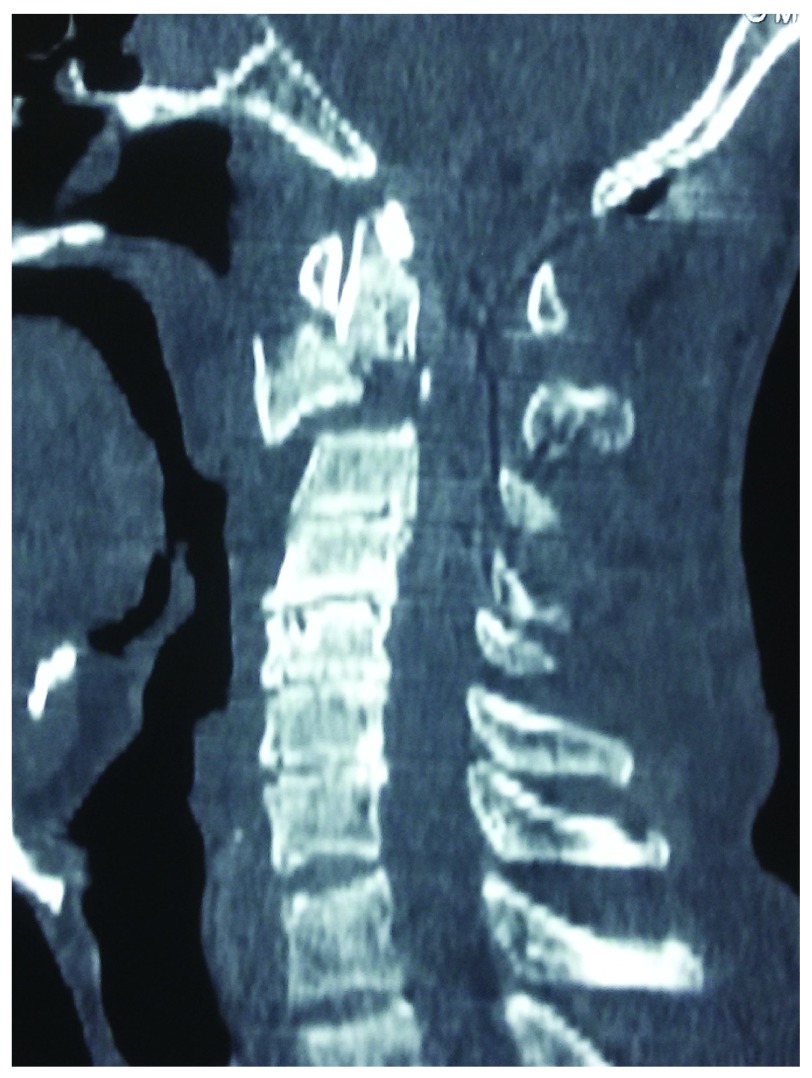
CT spine showing good reduction of the posterior and the spino-laminar lines and normal canal.
